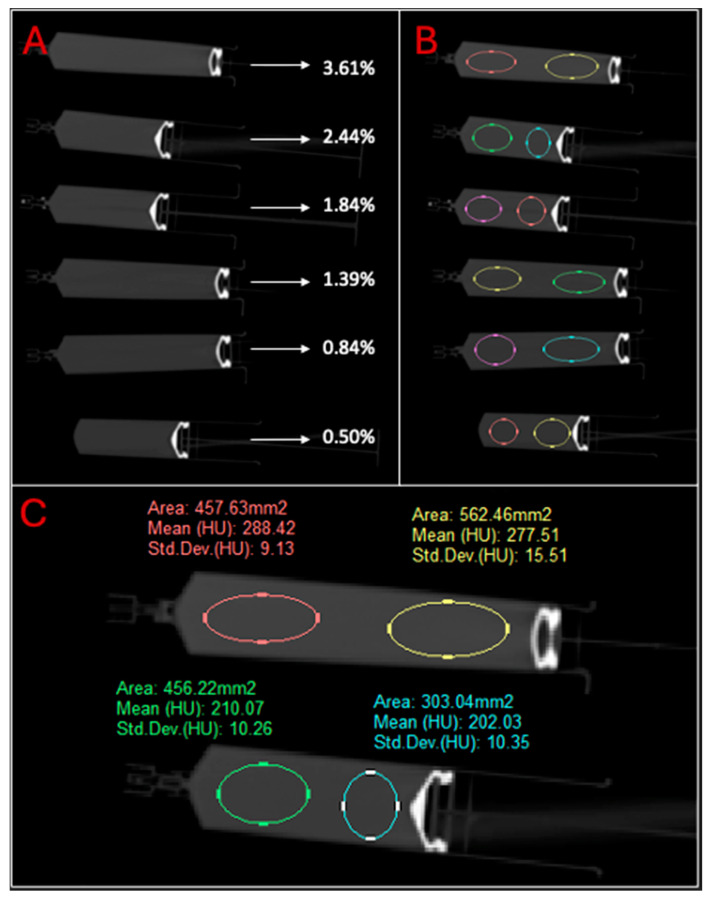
(A) Coronal CT image of contrast solution syringe gantry arrangement with concentration expressed as *v*/*v* percentage. (B) ROI placement within each syringe. (C) ROI placement for 3.61% and 2.44% contrast solution syringes shown with colored circles and corresponding labels with attenuation measurements.
